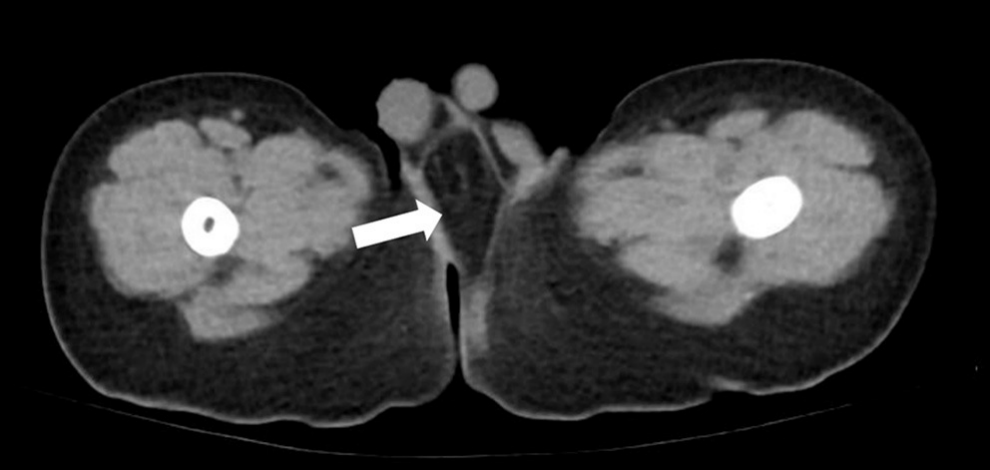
Axial scan on CT showed a low-density mass (white arrow) that had a clear margin at the perineum behind the scrotum (case 7).
